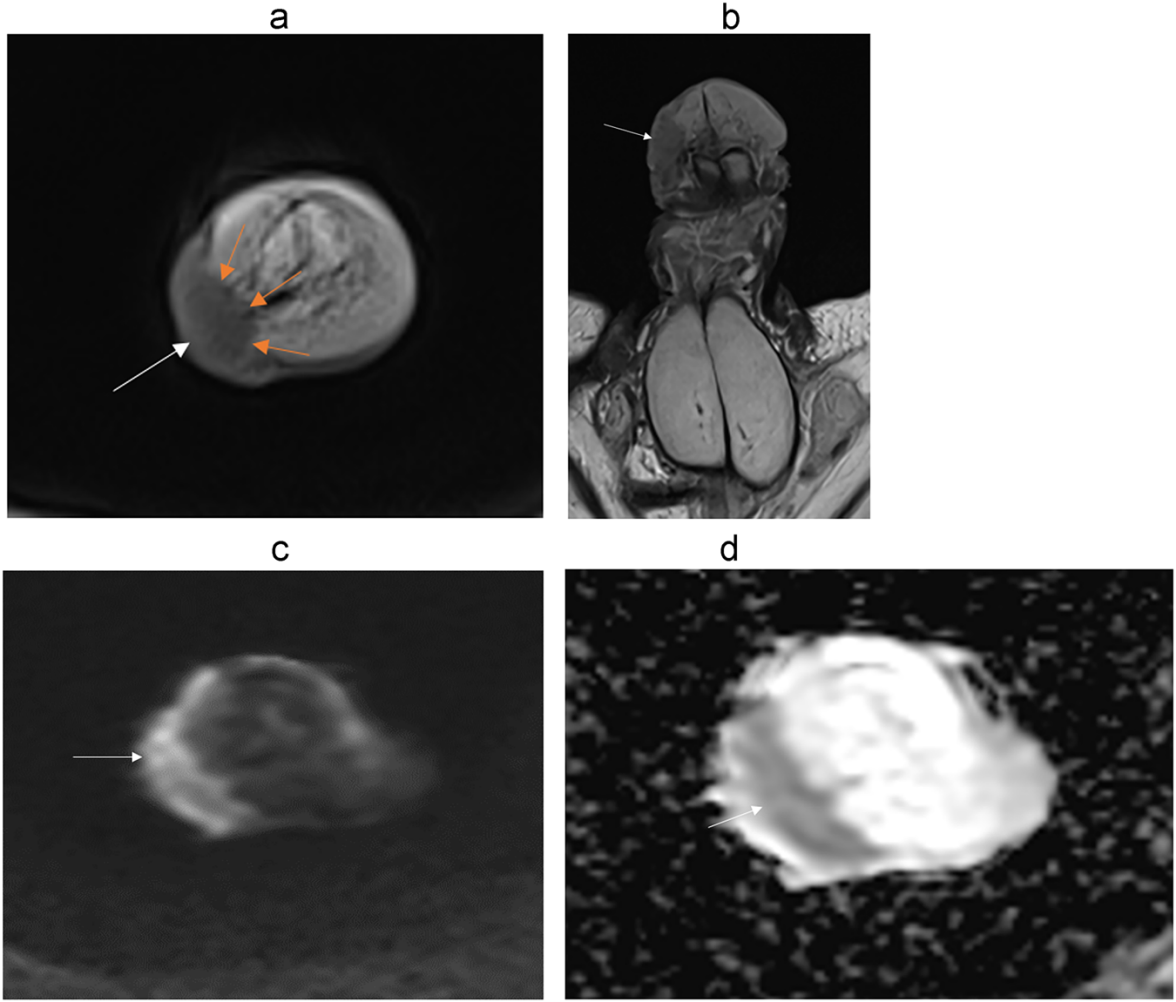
Eighty-three-year-old patient, referred for a cT2 lesion on the right hemi-glans. a Axial, (b) coronal T2-W MR images show a tissue lesion (with arrow) located on the right lateral ventral surface of the glans, which is hypointense to the corpora, invading the corpus spongiosum (orange arrows). c Axial DWI and (d) ADC map show intense diffusion restriction. Patient underwent a partial amputation revealing a non-HPV-related epidermoid carcinoma of the papillary type, infiltrating the corpus spongiosum to a maximum height of 0.9 cm. Stage pT2N0
